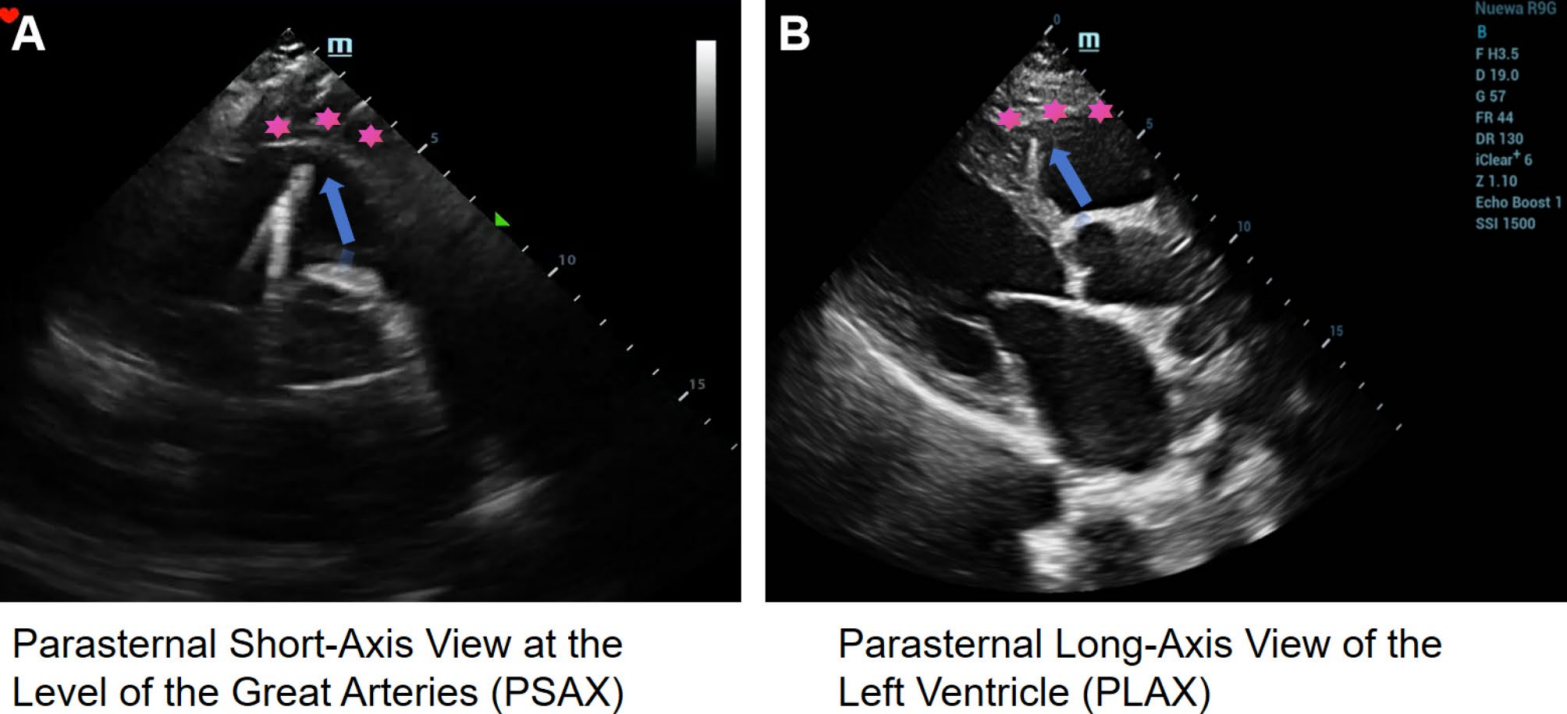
Panel A: Parasternal short-axis view of the great arteries. Panel B: Parasternal long-axis view of the left ventricle. In both images, the blue arrows indicate the ventricular lead, while the star symbol marks the free wall of the right ventricle. The images demonstrate that the right ventricular lead is positioned at the free wall of the right ventricle, which is identified as the origin of the ventricular tachycardia
